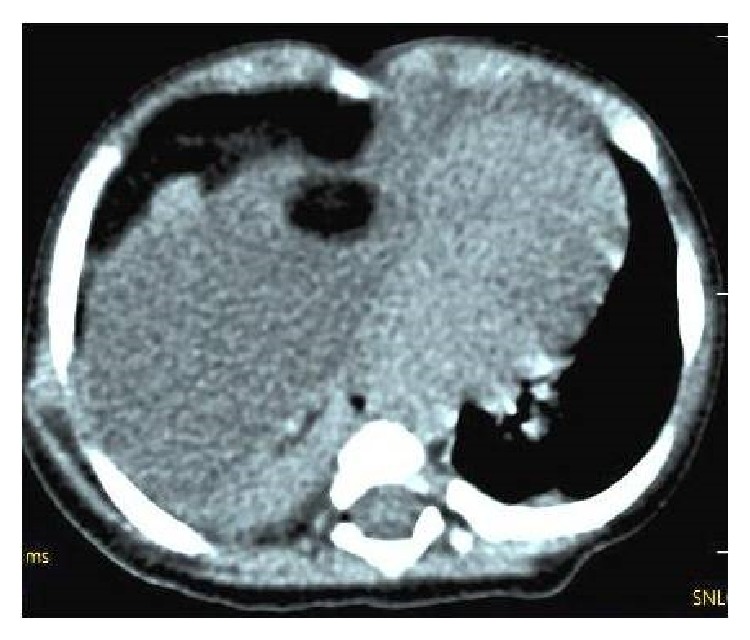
A CT image of the thorax.
